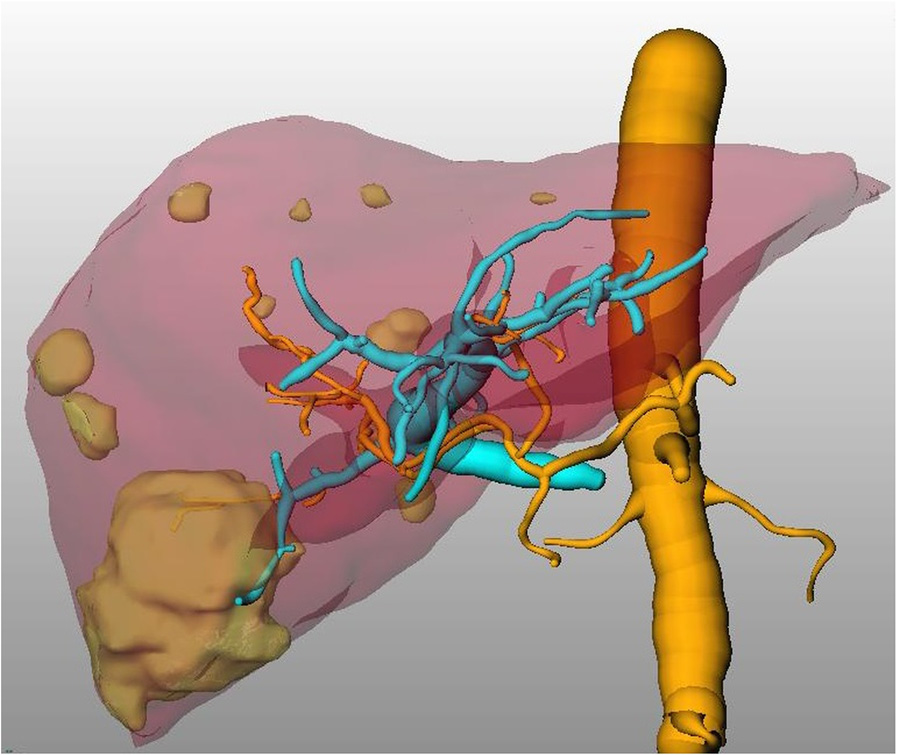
Three-dimensional reconstructed CT showing the masses with the aorta, hepatic artery, and portal vein.
